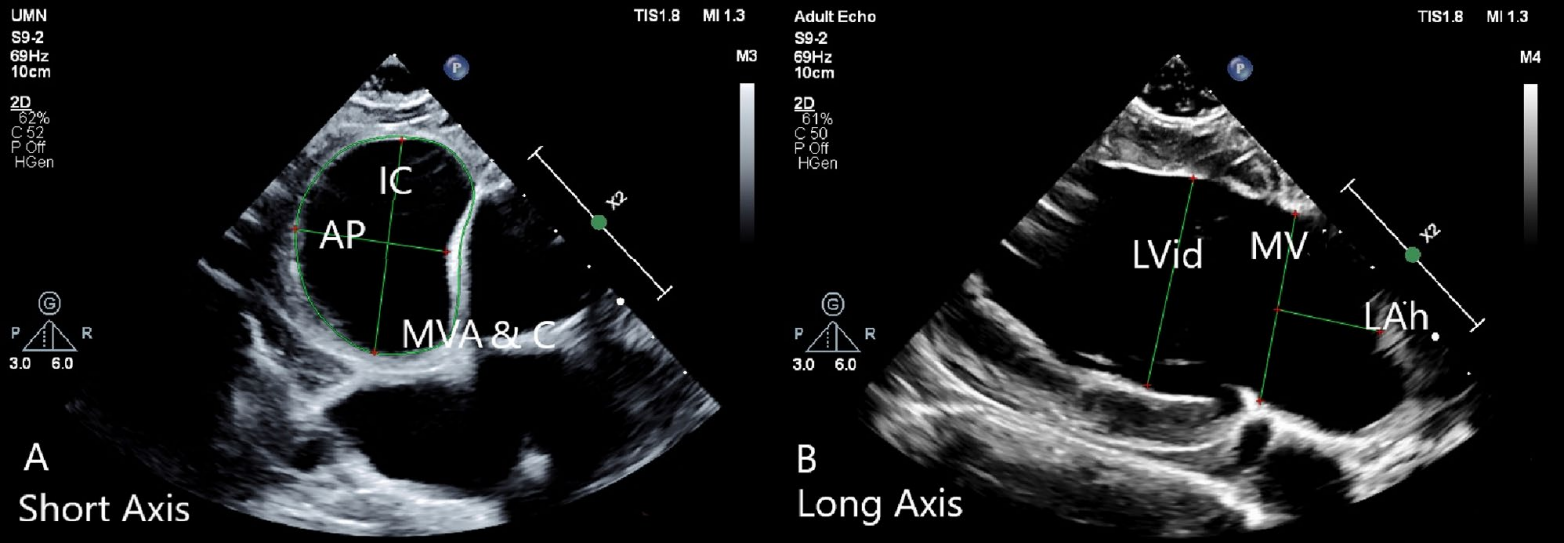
The mitral valve and left heart structures as viewed by echocardiography in short and long axis windows. Image (A) presents the short axis view of the mitral valve, with the inter-commissural (IC) and anterior–posterior (AP) dimensions, and mitral valve area and circumference (MVA & C) tracings indicated by green lines. Image (B) presents the long axis of the mitral valve, left ventricle and atrium. The left atrial height (LAh), mitral valve annular location (MV) and left ventricle inner diameter (LVid) are indicated by green lines.
